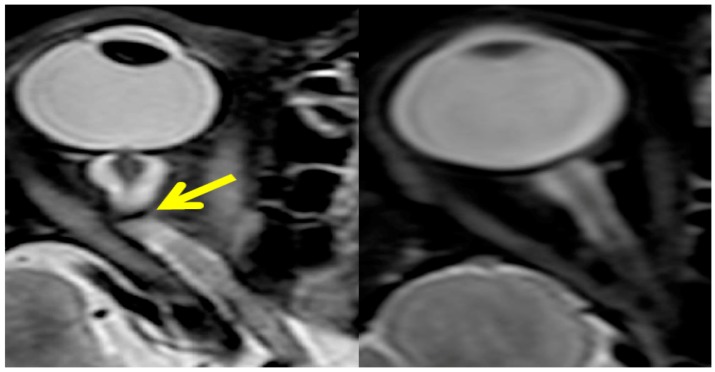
The postflight magnetic resonance image on the left shows that optic nerve kinking is present, while the control orbit on the right does not exhibit any tortuosity in the nerve. Source: National Aeronautics and Space Administration.
